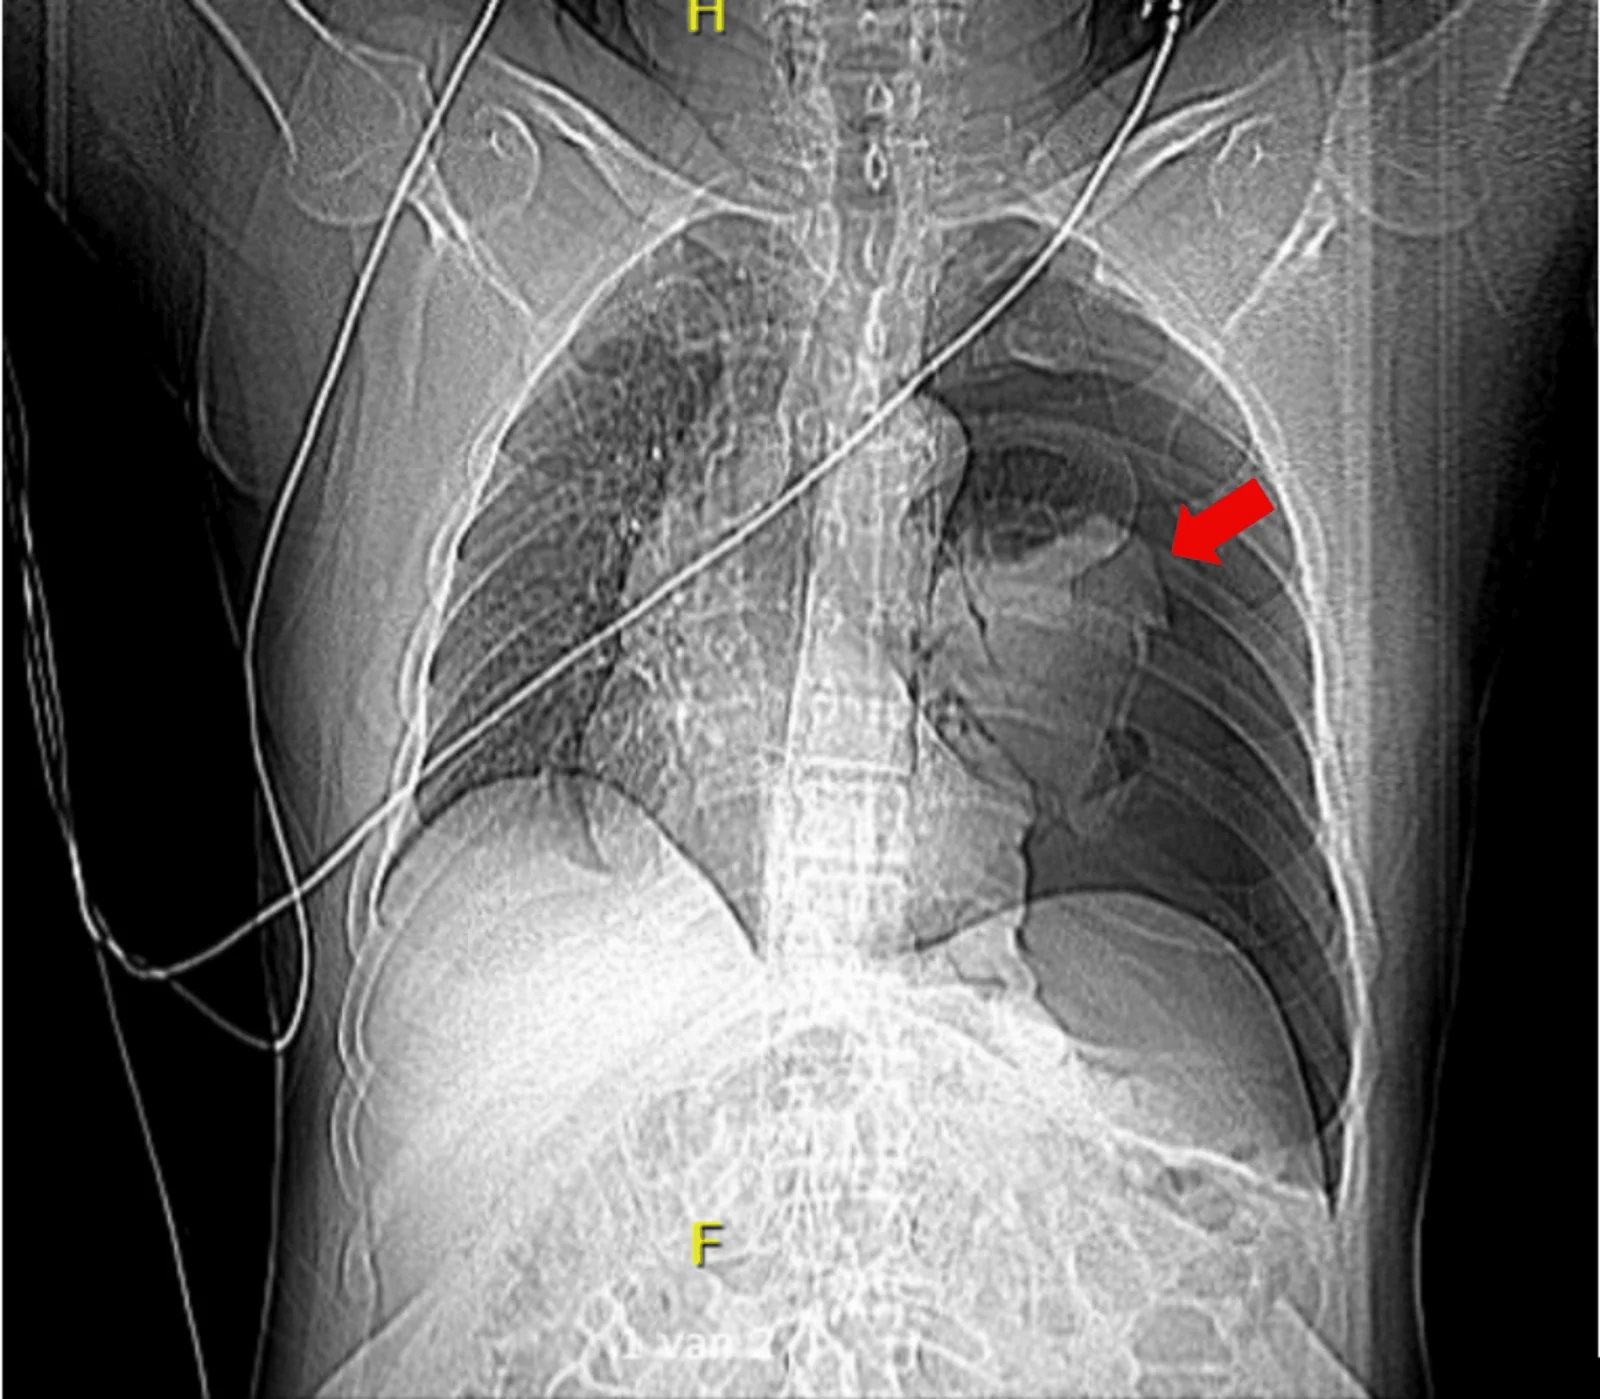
Initial CT scan demonstrating left-sided hydropneumothorax with collapse of the left lung. The red arrow indicates the collapsed left lung. CT: computed tomography
